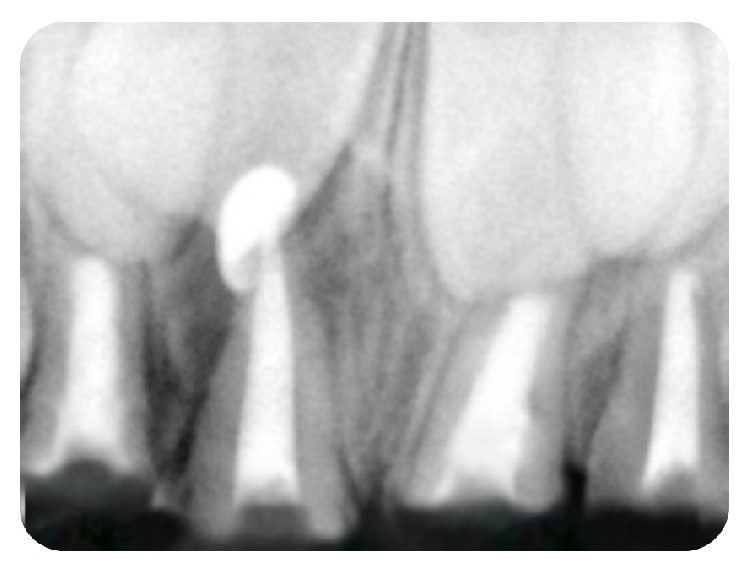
Postobturation radiograph in relation to 51, 52, 61, and 62.
